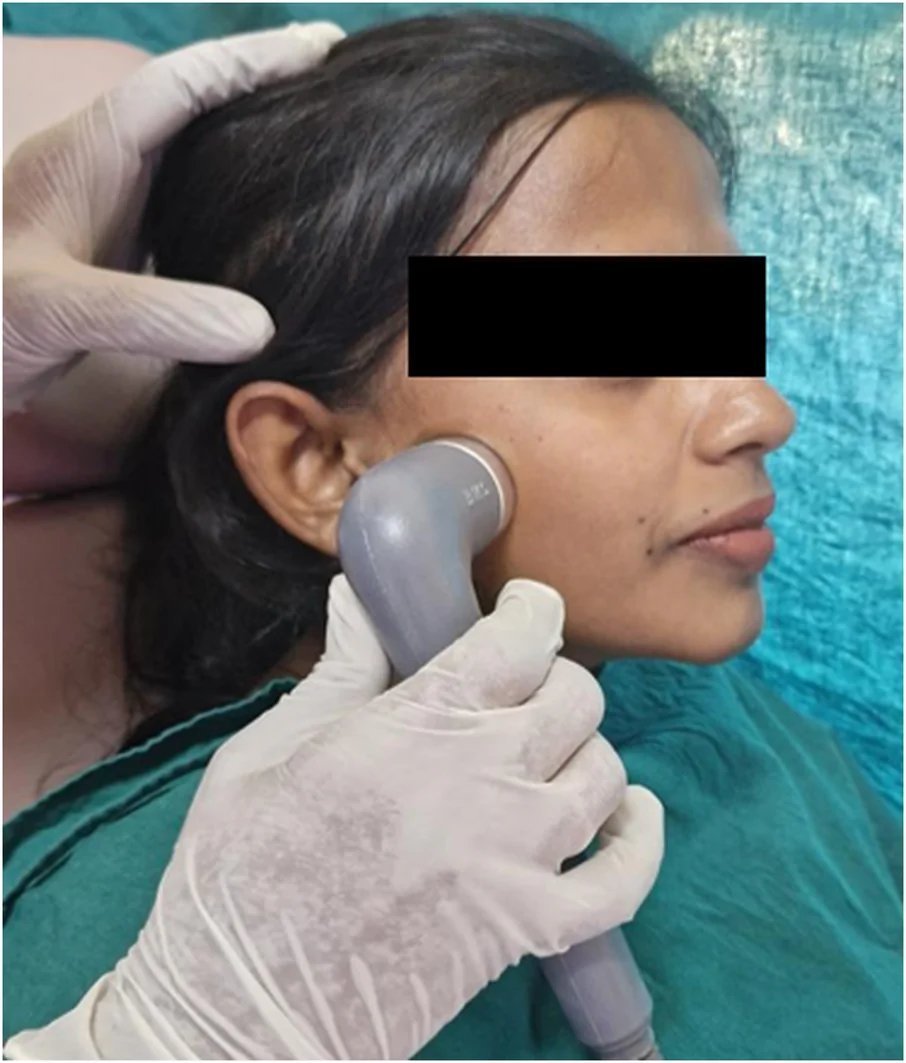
Participant during ultrasound therapy.
